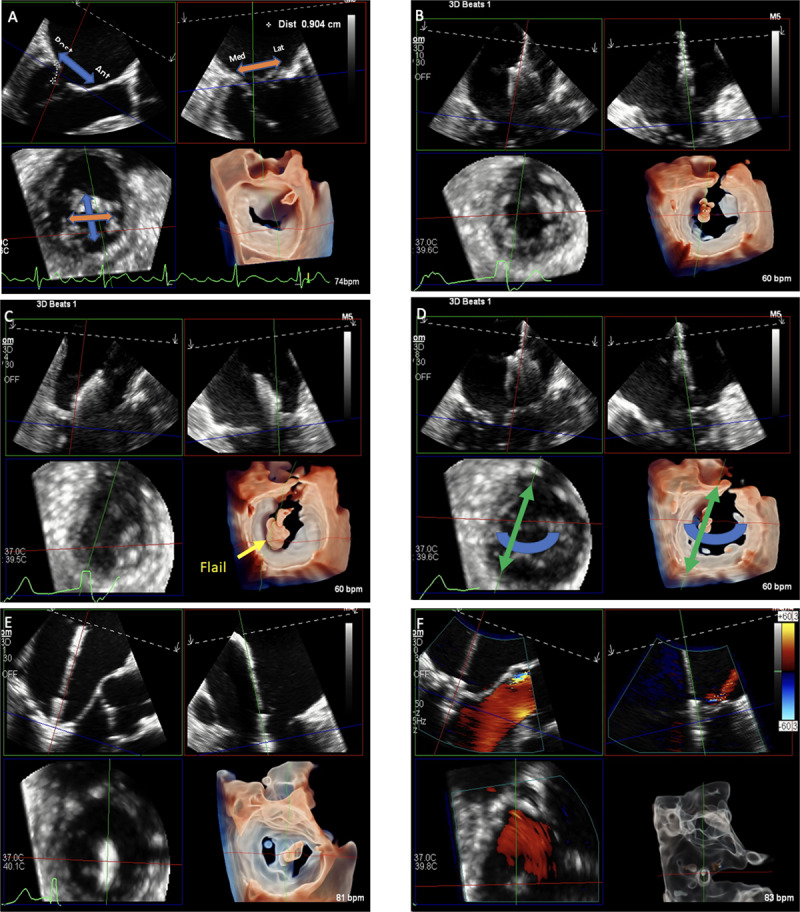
Multiplanar reconstruction (MPR) of the mitral valve using transesophageal echocardiography for assessment and procedural guidance. Multiplanar assessment of the mitral valve (A) is done by visualizing the long-axis view (top left plane) of the mitral valve on which anterior and posterior locations could be localized, the bi-commissural (top right) plane where medial and lateral coordinates can be determined; both of these planes will determine the small axis plane (bottom left) and its 3D reconstruction (bottom right). During the procedure, real-time MPR allows (B) visualization and adjustment of the device trajectory, (C) positioning of the device according to disease location, (D) orientation of device for optimal grasping, (E) advancement of the device and grasping, and (F) evaluation of device attachment and residual disease.
